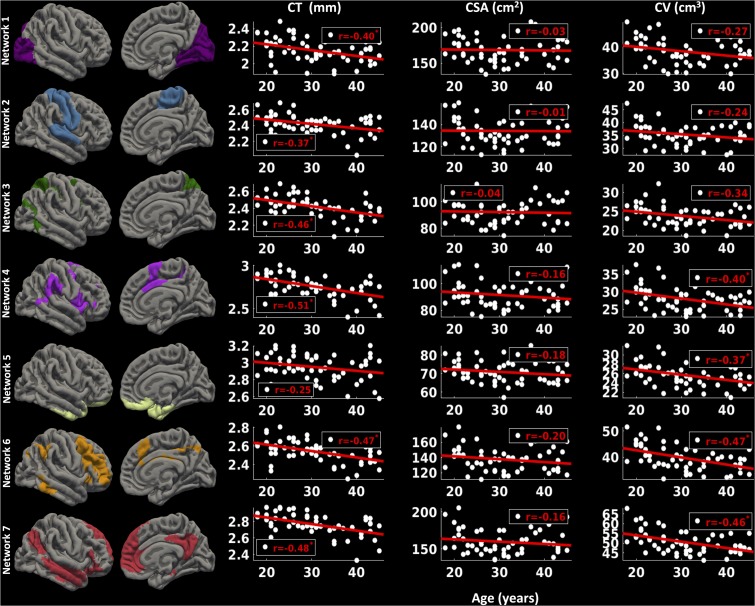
Network-wise age-related changes in cortical measures for right hemisphere. Linear plots showing significant reduction in cortical thickness (CT in mm) (^∗^*p* < 0.05, corrected for multiple comparisons), non-significant reduction in cortical surface area (CSA in cm^2^) and significant or trend toward significant reduction in cortical volume (CV in cm^3^) (^∗^*p* < 0.05, corrected for multiple comparisons) for seven functional networks on right hemisphere.
